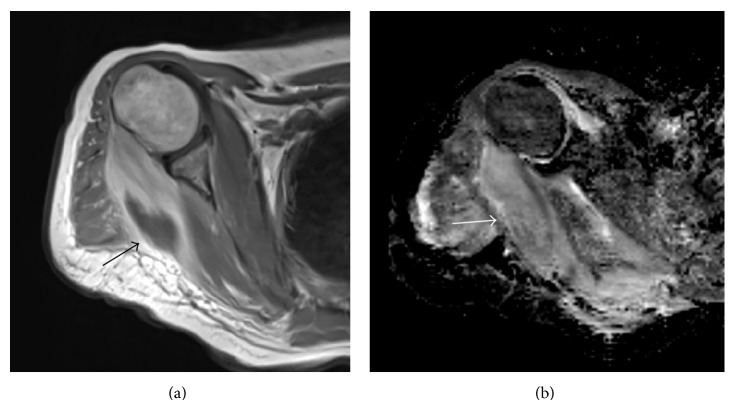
(a)* Axial MRI* image of the* Rhizopus* abscess. Contrast enhanced T1W image shows thickening of the teres minor and major muscles with heterogeneous enhancement and hypointense central area of necrosis suggestive of abscess (black arrow). (b) High signal in the ADC ap of DW sequence (*b* = 600 s/mm^2^ in the diffusion (white arrow) (MRI: magnetic resonance imaging; T1W: t1 weighted; ADC: apparent diffusion coefficient; DW: diffusion-weighted)).
